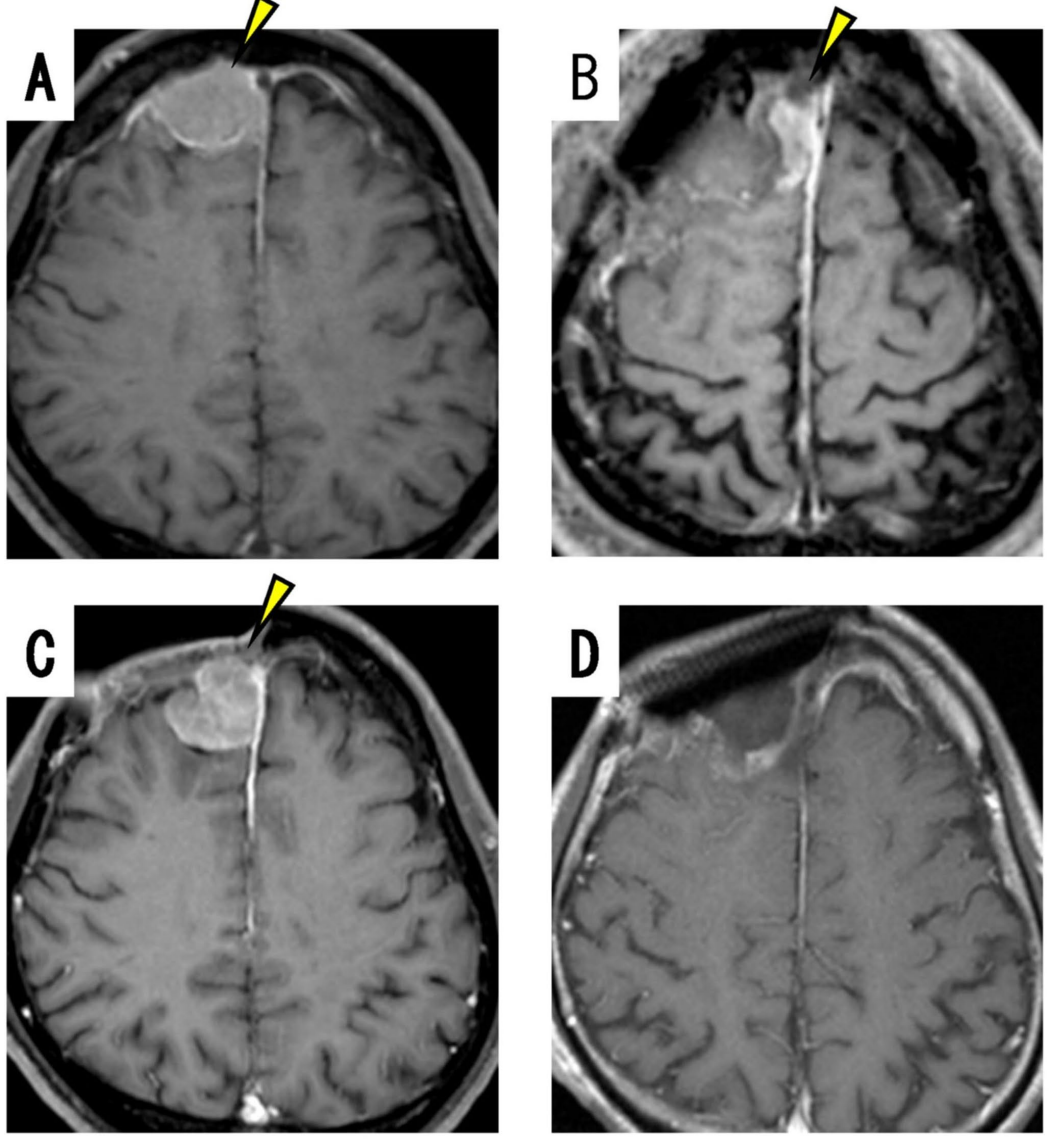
Case 2 (man in his early fifties): grade 3 meningioma in the right parasagittal sinus without prior RT. Gadolinium-enhanced brain MRI taken before (A) and 2 days after the initial operation (B) displaying the tumor in the right parasagittal sinus (A: yellow arrowhead) and the residual tumor (B: yellow arrowhead). (C) Follow-up MRI taken 2 years after the operation displaying recurrence at the original site (yellow arrowhead). (D) Gadolinium-enhanced brain MRI taken 3 weeks after PDT displaying complete removal of the tumor
